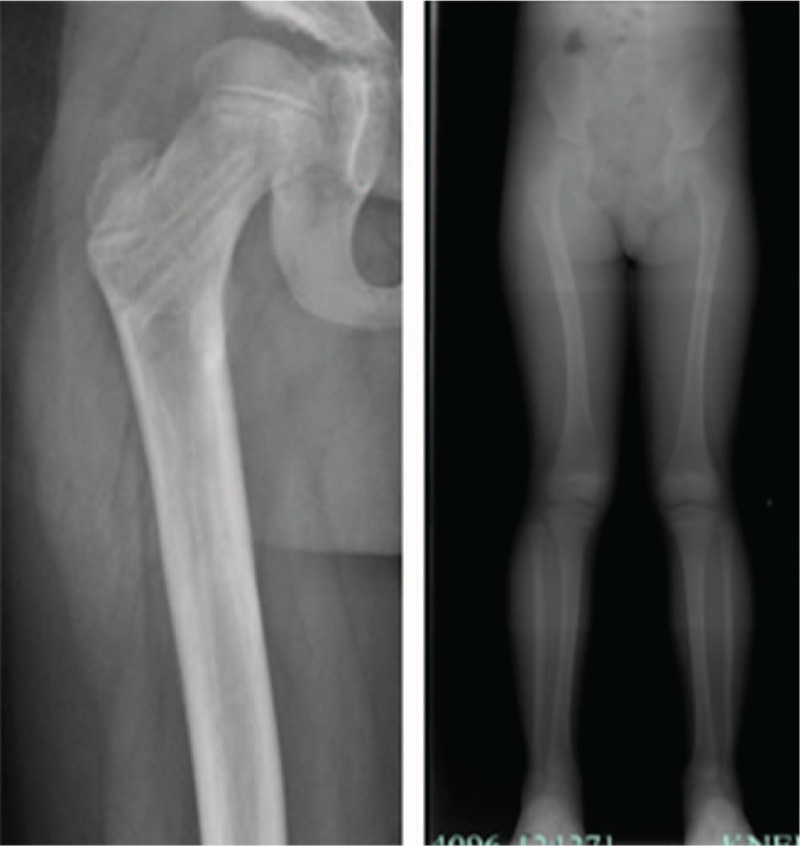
The above pictures are the right femur X-ray films after removal of internal fixation 1 year after operation, and the full length of the lower limbs after removal of internal fixation for 14 months.
